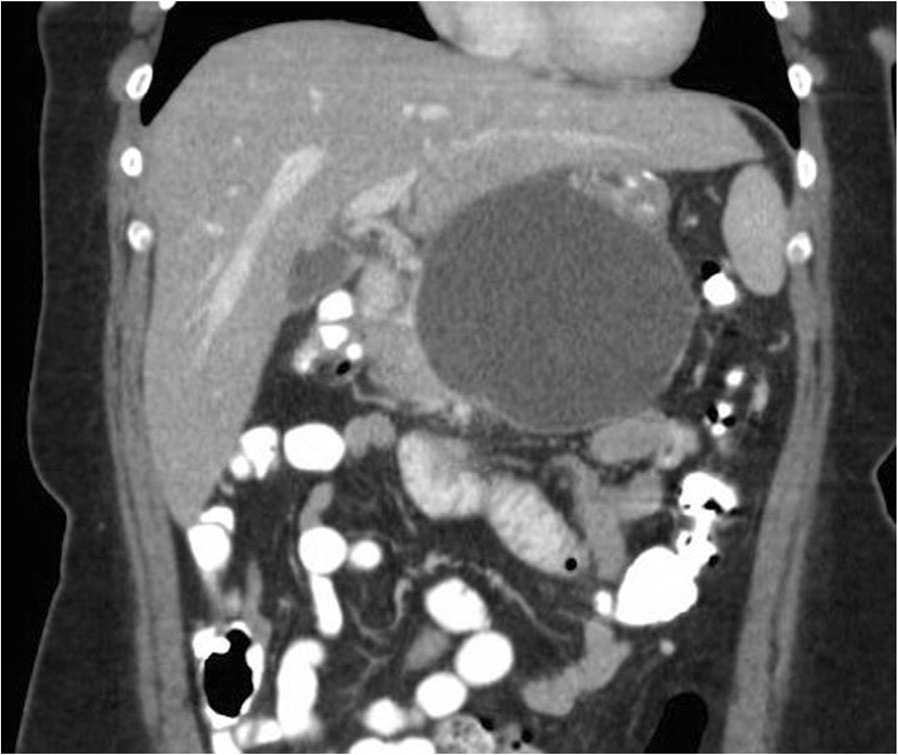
WOPN imaging (coronal CT image)
